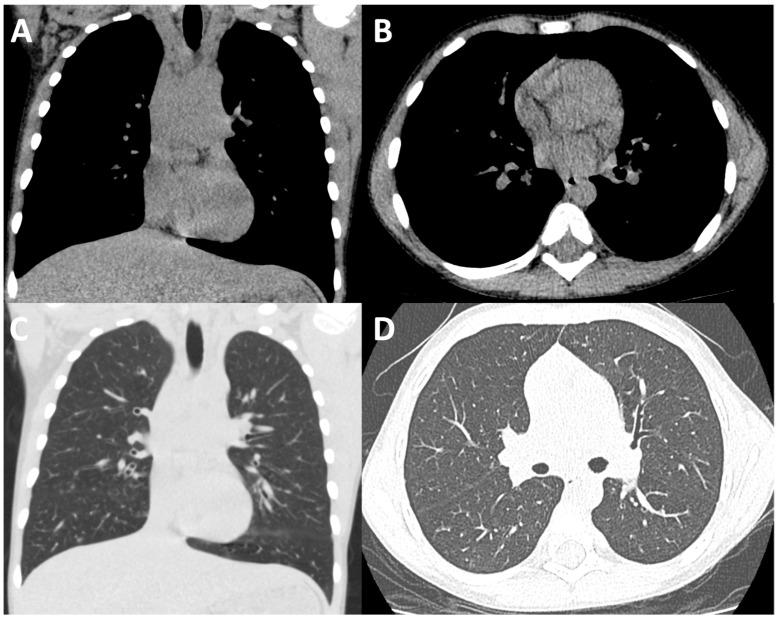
Conventional-dose CT of the thorax. Coronally (A,C) and axially reconstructed (B,D). Conventional-dose CT of the thorax images in soft tissue (A,B) and lung windows (C,D) in a paediatric male CF patient performed during an acute hospital admission. The dose length product (DLP) for this examination was 39.77 mGy*cm.
